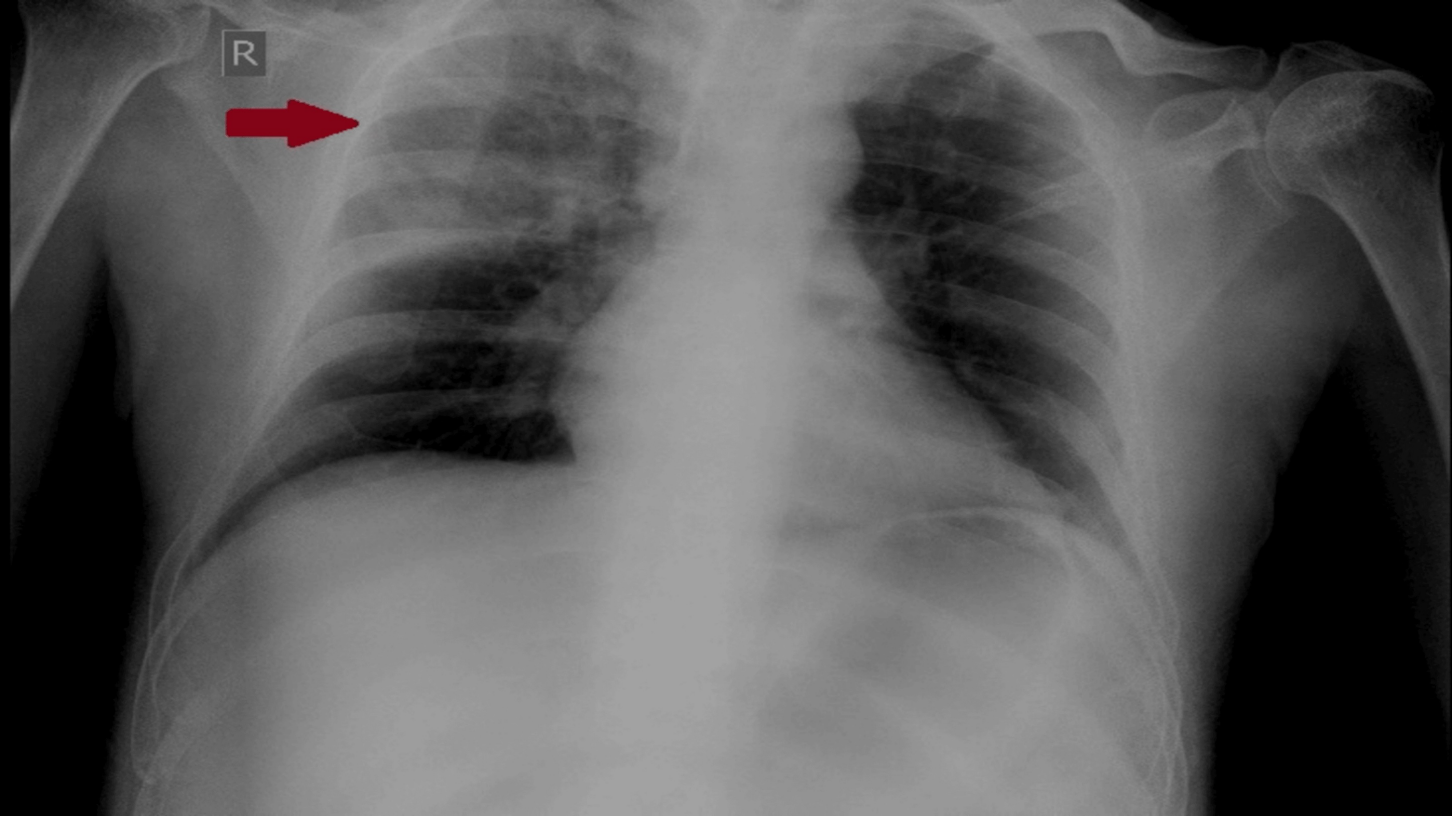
Chest X-ray showing right upper lobe opacity as depicted by the red arrow
